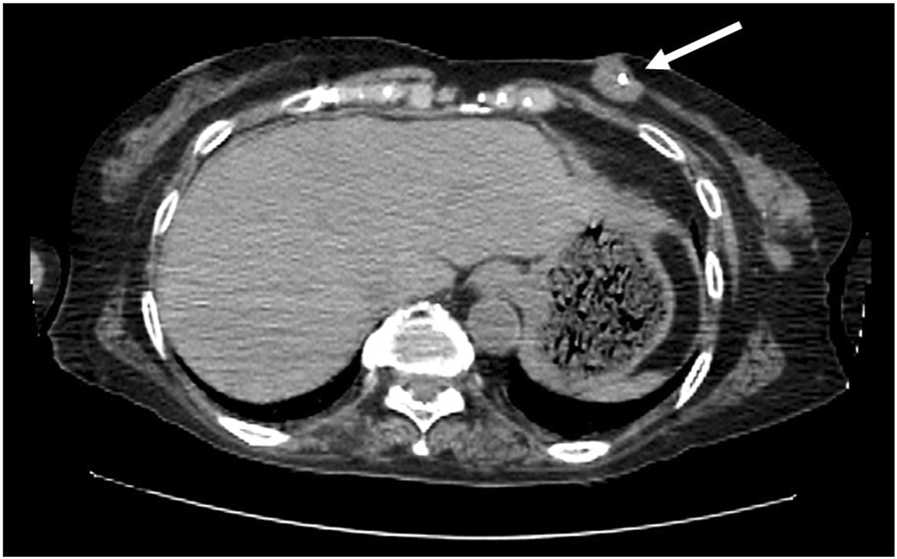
A CT scan showed that the tumor involved the VP shunt
